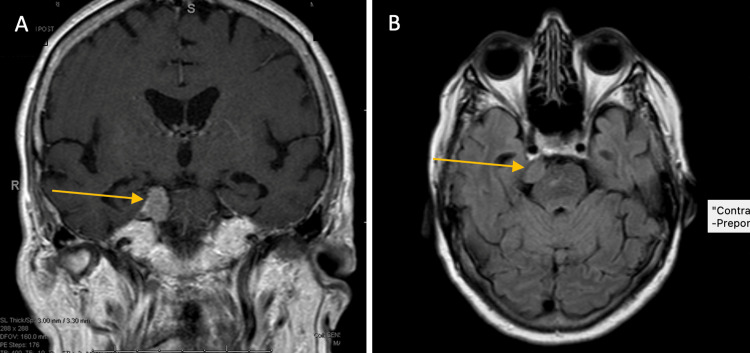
Surveillance brain MRI demonstrating stable cerebellopontine angle meningioma without edema (A) Coronal T1-weighted contrast-enhanced MRI demonstrated treated right cerebellopontine angle meningioma (yellow arrow), unchanged from pre-treatment. (B) Axial fluid-attenuated inversion recovery (FLAIR) MRI demonstrating no hyperintense signal in association with the treated meningioma (yellow arrow).
